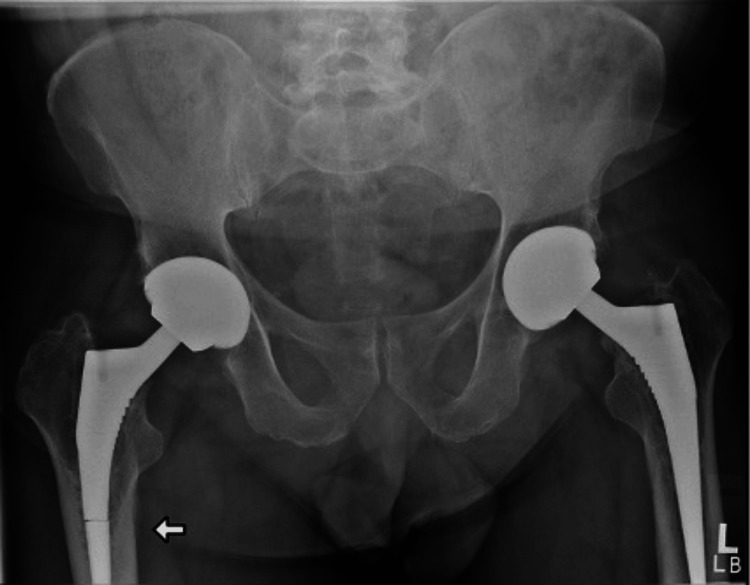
X-ray (anteroposterior view) of bilateral hip joints showing a femoral stem fracture on the right side at presentation to the clinic
